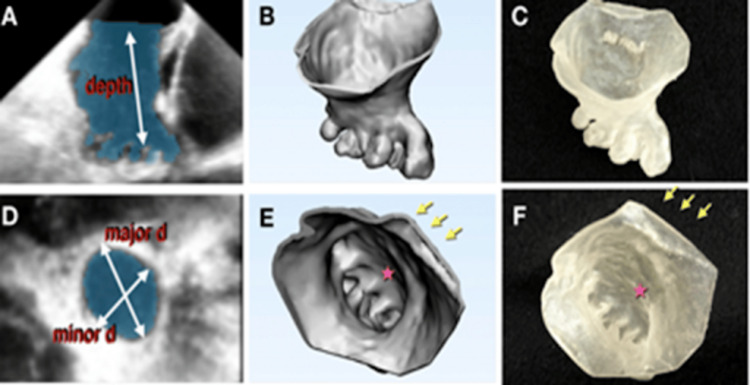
Progression of taking (a) echocardiography images to (b) 3D models to (c) 3D printouts. The same progression is depicted in (d) echocardiography images to (e) 3D models to (f) 3D printouts in a superior view Adapted/reproduced from Fan et al. [11], with open access permissions under the Creative Commons contribution agreement
